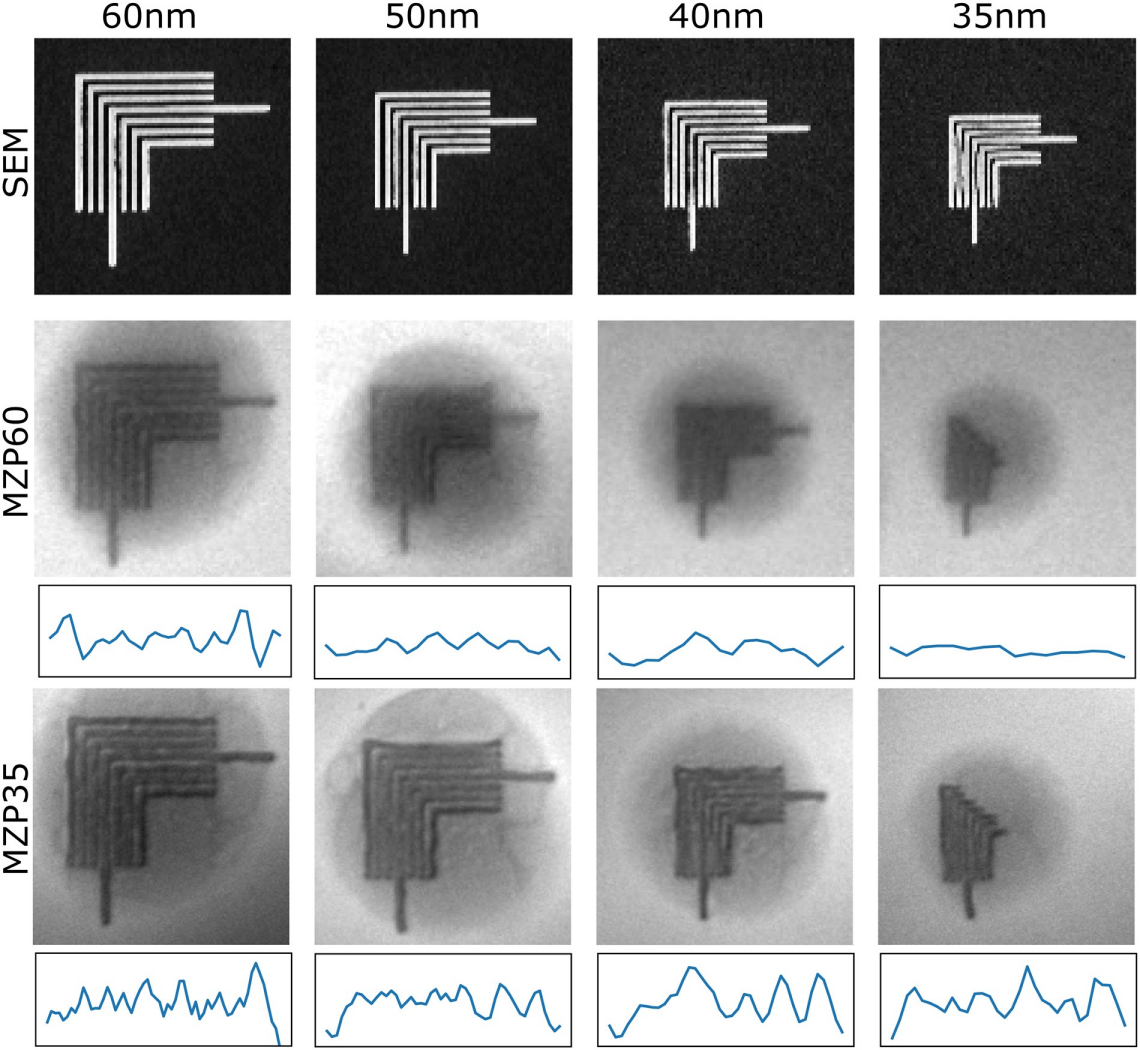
Characterization of 60 nm and 35 nm zone width objectives. Characterization of SXT objectives 60 nm and 35 nm outermost zone width. Top: SEM images of the fabricated test patterns, selected randomly on the supporting membrane. Middle: X-ray projection images of test patterns with 60 nm MZP, and bottom: the same structures visualized with 35 nm MZP. The field of view of x-ray projection images is 1.4 μm x1.4 μm. Below each x-ray projection image is a line profile through the test pattern. A spherical background on test patterns is due to remaining photoresist.
